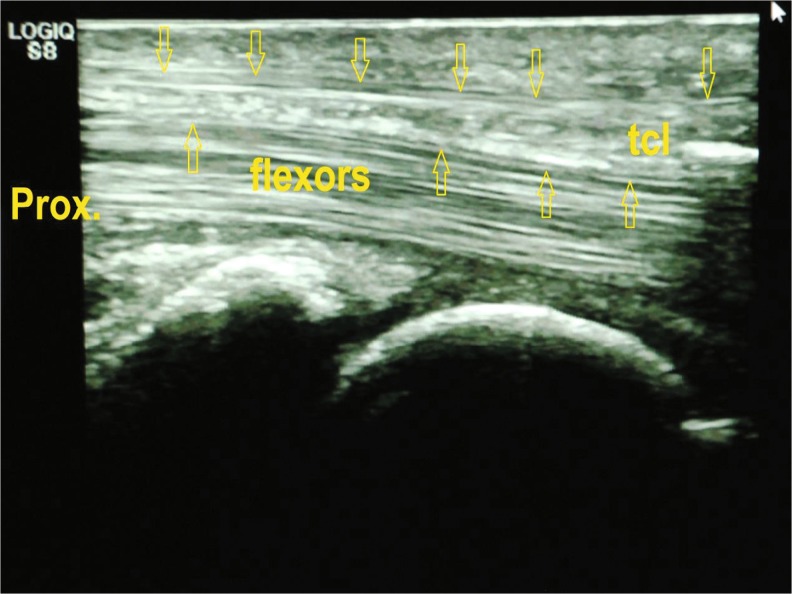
Thread in loop in ultrasound view
